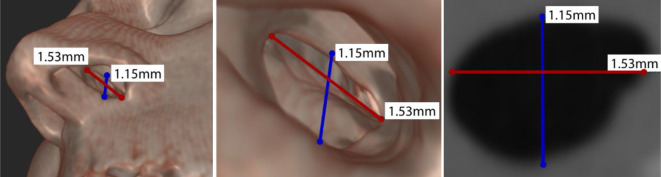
Computed tomography scan: determination of nasal septum perforation expansion in horizontal and vertical direction
